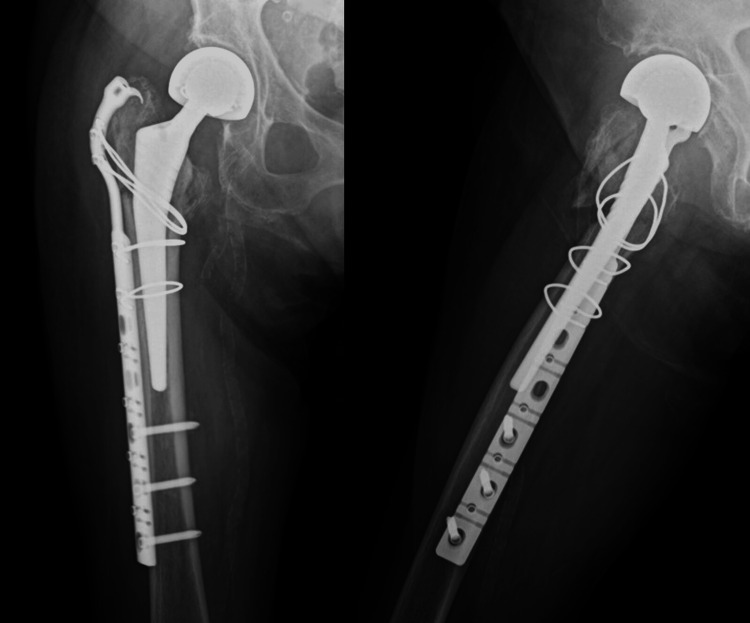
Postoperative radiographs of case 2 ORIF with plate fixation and cerclage wiring was performed. ORIF: open reduction and internal fixation.
